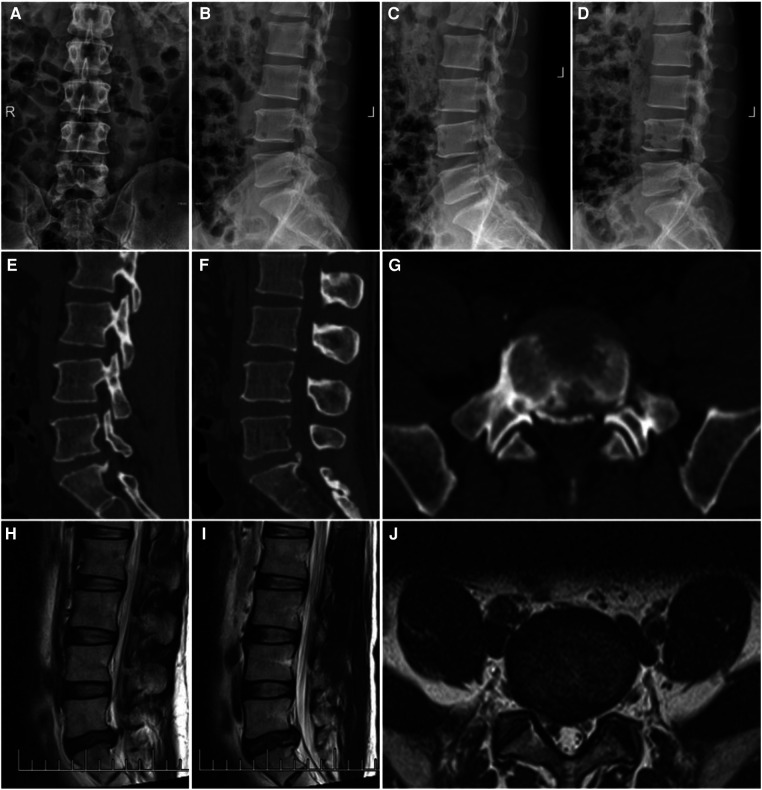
A 44-year-old man with LDH linked with PRAS was treated with PEID; the symptoms were mainly pain in the right lower limb. (A,B) CT scan of the lower lumbar spine: the detached bone fragment is shown to protrude into the posterior margin of the vertebral body and (C,D) MRI of the lumbar spine: the imaging findings were consistent with that of the CT scan.
